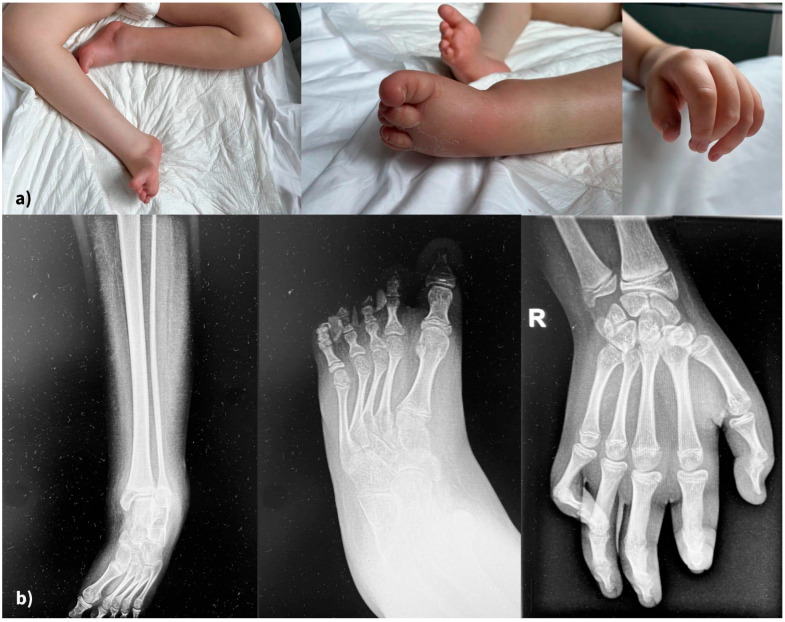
(a) Severe, irreversible limb deformities (clinical aspects), (b) X-rays of lower and upper limbs.
